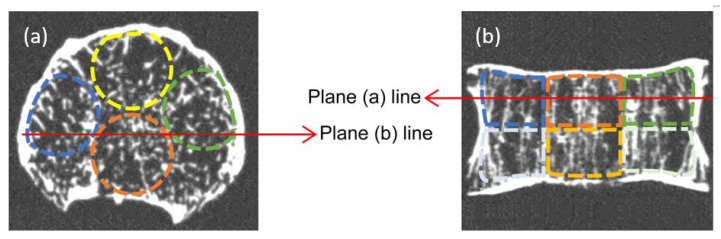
Volume of interest determination in cancellous bone. (a) Representative transverse image. (b) Coronal image. Both images are obtained by quarter-detector computed tomography. In the transverse direction, there were the following four regions: the ventral and dorsal directions, and the left and right directions. In the coronal image, eight cylindrical regions were set, which were divided into two from the center of the vertebral body in the cranial and caudal directions.
